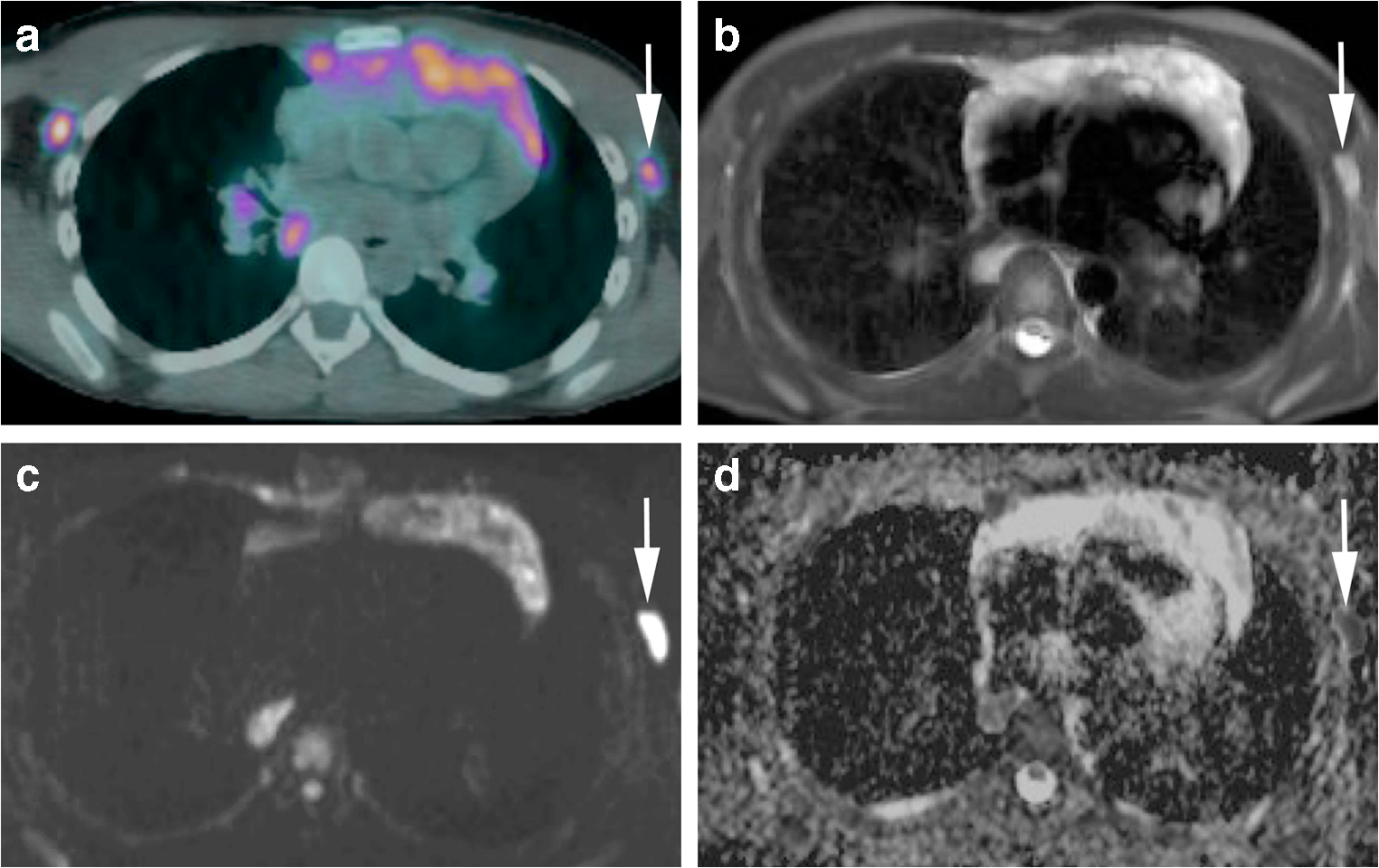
Images in a 15-year-old boy with Hodgkin lymphoma. a Axial ^18^flourine-2-fluoro-2-deoxyglucose (FDG) positron emission tomography (PET)/CT shows a positive left axillary node (*arrow*). b Axial short tau inversion recovery half-Fourier acquisition single-shot turbo-spin-echo (repetition time/echo time [TR/TE] = 800/60 ms) MR image demonstrates an 8-mm short-axis-diameter left axillary node (*arrow*). c Axial diffusion-weighted imaging (DWI; b=500 s/mm^2^; TR/TE=4,900/66 ms) demonstrates an 8-mm short-axis-diameter left axillary node. These findings would result in false-negative nodal classification according to the threshold of short-axis diameter ≥10 mm. d Apparent diffusion coefficient (ADC) map demonstrates the same left axillary node (*arrow*) with an ADC of 0.8×10^−3^ mm^2^ s^−1^. The node was deemed positive on whole-body MRI using the combined short-axis diameter ≥10 mm and ADC cut-off of <1.15×10^−3^ mm^2^ s^−1^ classification — a true-positive result compared with using the FDG PET/CT reference
